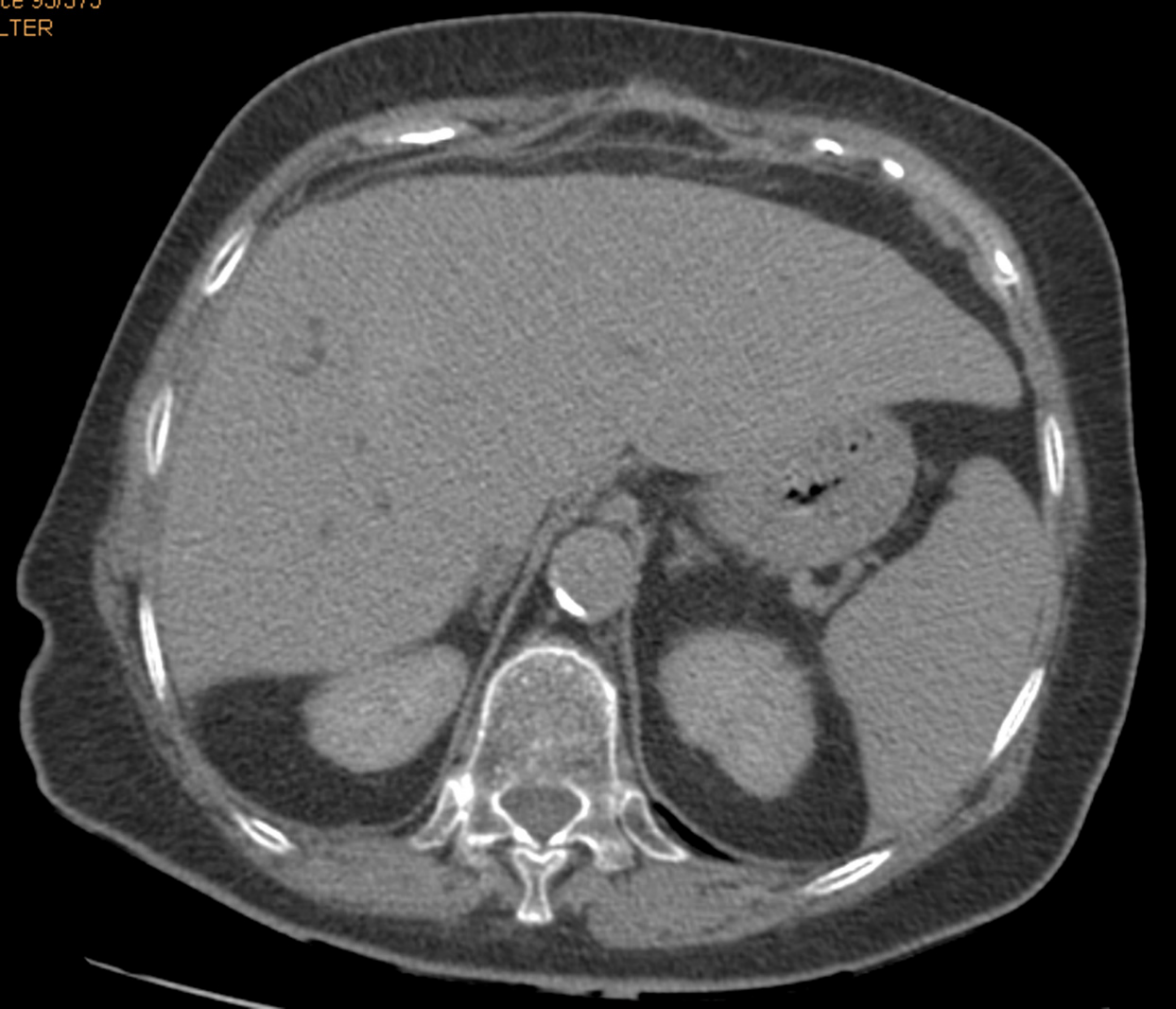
CT scan 2 months postoperatively showing a hypertrophied right liver and no evidence of any intra‐abdominal collections.
